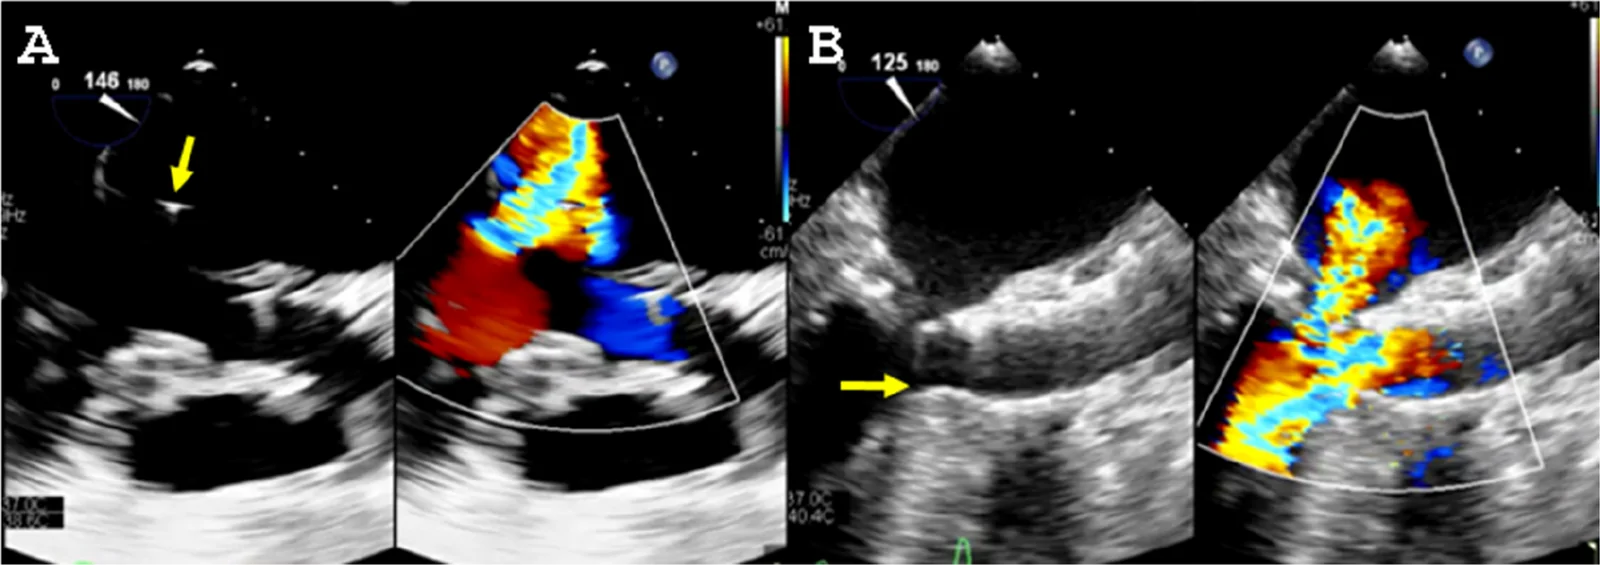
Detection of new or worsening mitral regurgitation (MR) during transcatheter aortic valve implantation (TAVI) procedure. A Significant MR jet due to guidewire (arrow) interfering with subvalvular apparatus and mitral leaflet motion. B Systolic anterior motion of anterior mitral leaflet giving rise to MR and dynamic left ventricular outflow tract obstruction post TAVI due to thick basal (sigmoid) septum (arrow) confirmed on intraprocedural transesophageal echocardiography imaging
